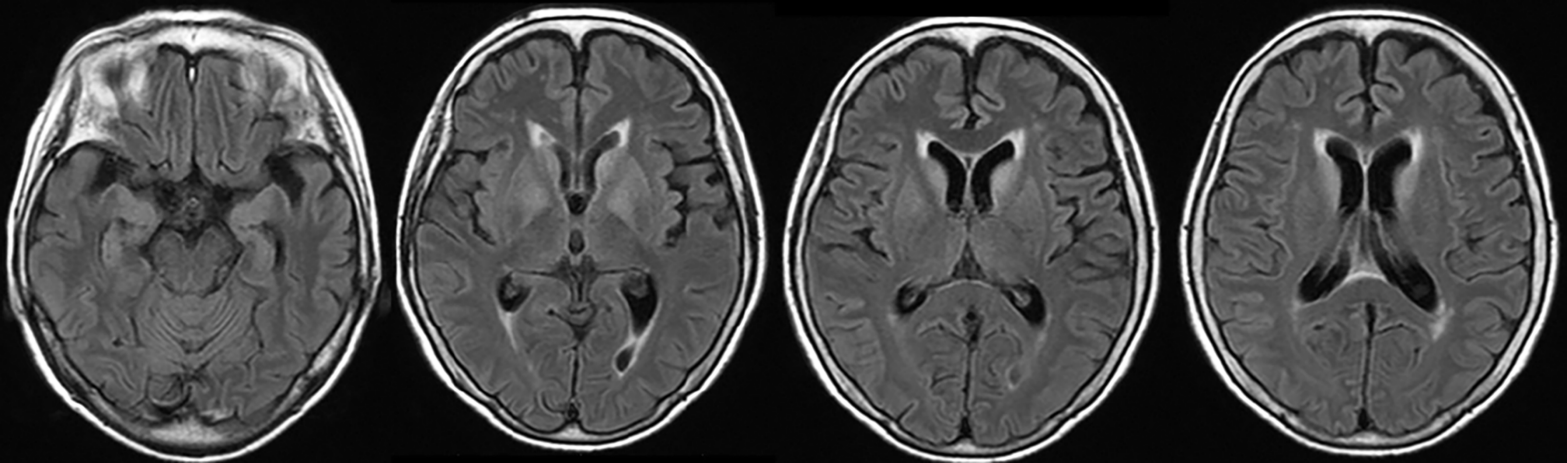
Brain MRI showed symmetric increased fluid-attenuated inversion recovery signals in the basal ganglia and medial temporal lobes.
